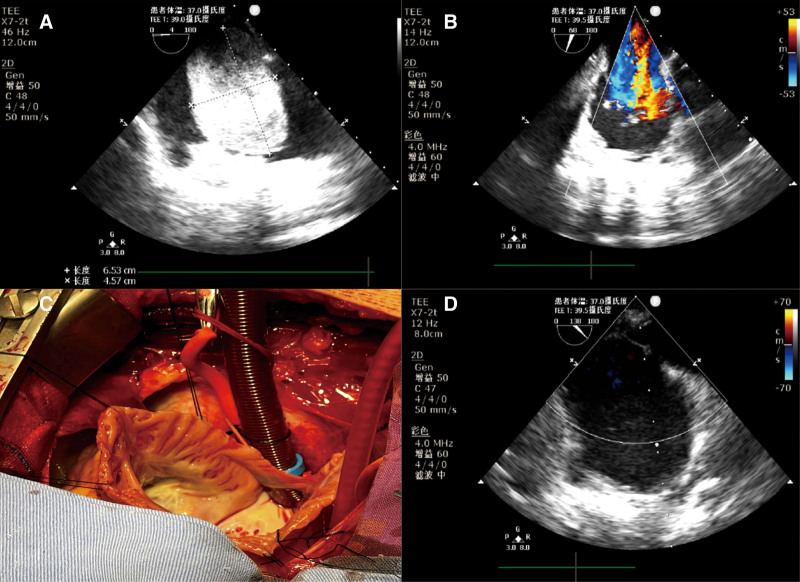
(A) Transthoracic echocardiography reveals a right atrial mass with inhomogeneous echo (65.3 mm × 45.7 mm). (B) Apical 4-chamber view with color flow mapping, preoperatively, showing tricuspid regurgitation. (C) Intraoperative photograph showing the myxoma been resected in the right atrium. (D) Apical 4-chamber view with color flow mapping, postoperatively, showing none tricuspid regurgitation.
